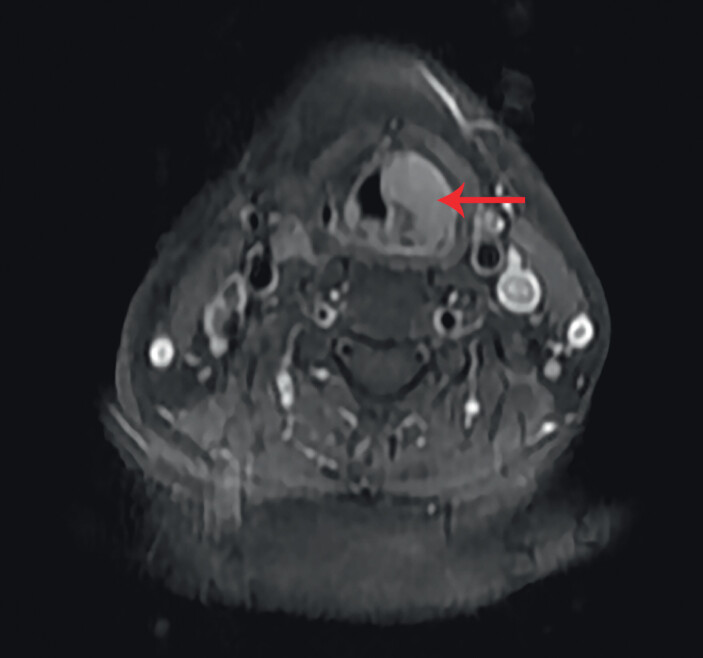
Magnetic resonance imaging revealed an abnormal signal (arrow) in the left piriform fossa involving the left aryepiglottic fold.
